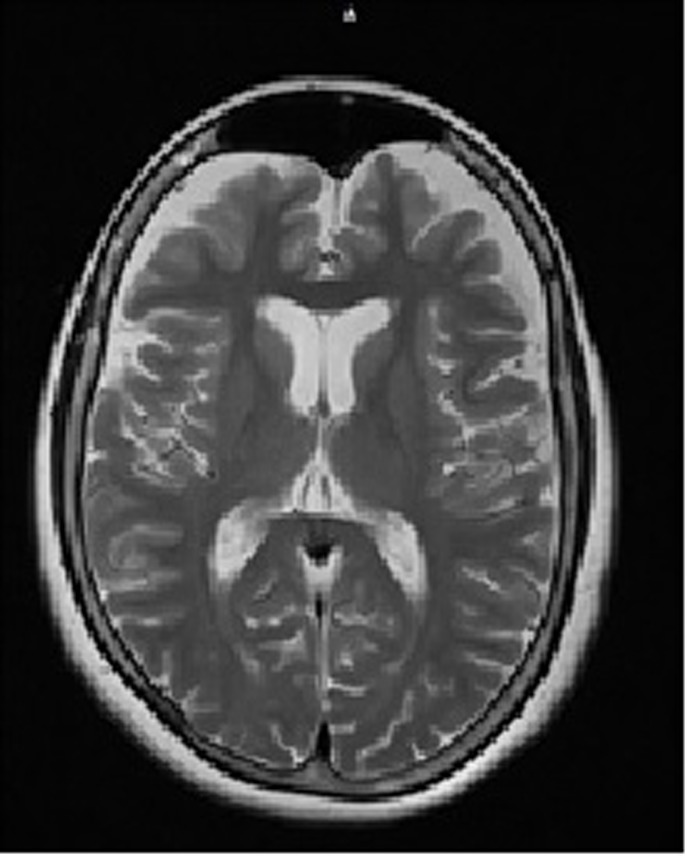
Magnetic resonance of the brain with gadolinium. Contrast-enhanced brain MRI shows marked cortical and subcortical atrophy in the frontal region plus dilation of the ventricular system.
